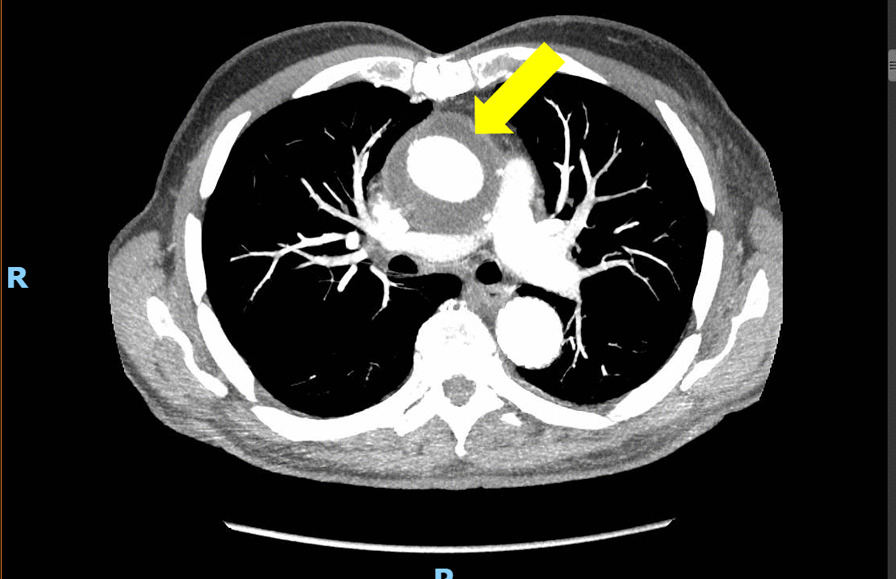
Computed tomography angiogram demonstrating significant fluid collection surrounding the prosthetic ascending aorta (arrow)
